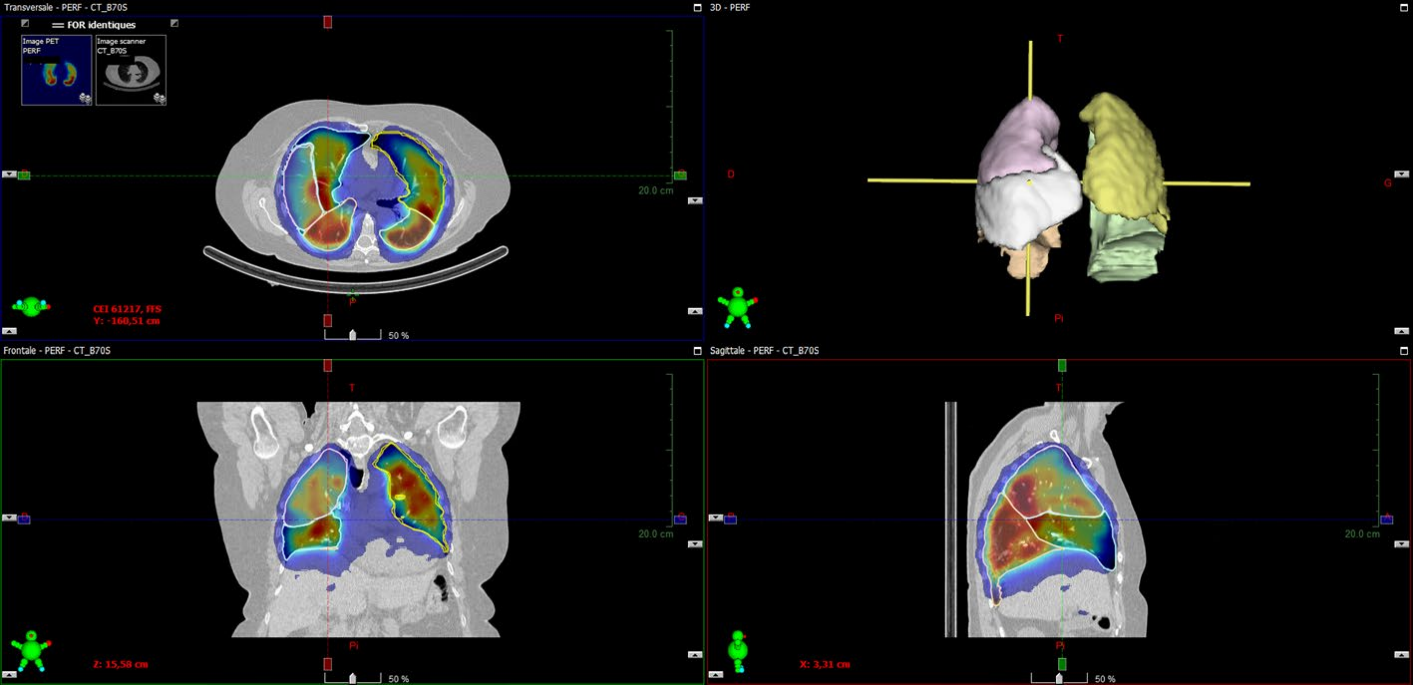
Manual lobar segmentation on the perfusion SPECT/CT dataset imported in Eclipse. The segmentation was conducted by an experienced nuclear physician. Representative data of a single patient (the same patient in Figs. 1 and 2) are shown
